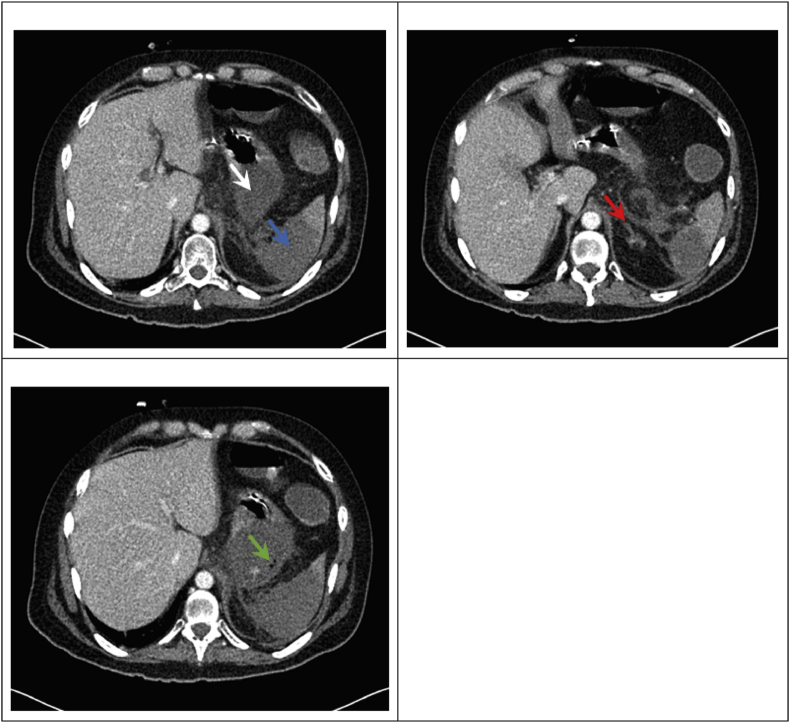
CT images during the venous phase. Subtotal infarct zone of the spleen (blue) with contrast enhancement only in an anterior segment of the spleen. Also partial infarction of the left adrenal gland (red), the gastric fundus and proximal gastric corpus (white) with adjacent free air bubbles (green) suggestive of gastric perforation. (For interpretation of the references to colour in this figure legend, the reader is referred to the Web version of this article.)
